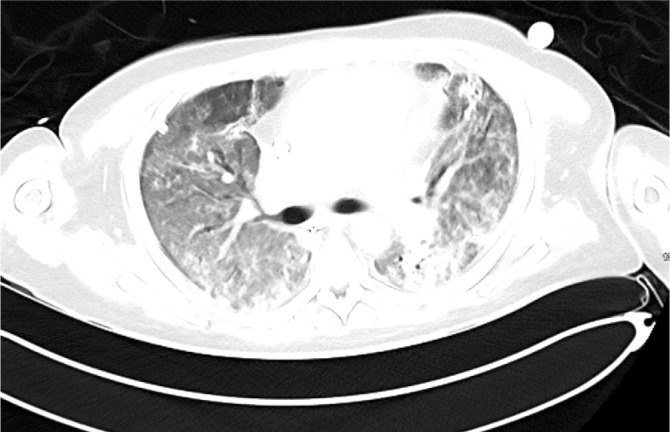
CT Thorax performed on Day 39 shortly after discontinuation of ECMO showed nodular and ground glass opacification which have improved significantly in the interim. There was some early traction bronchiectasis.
